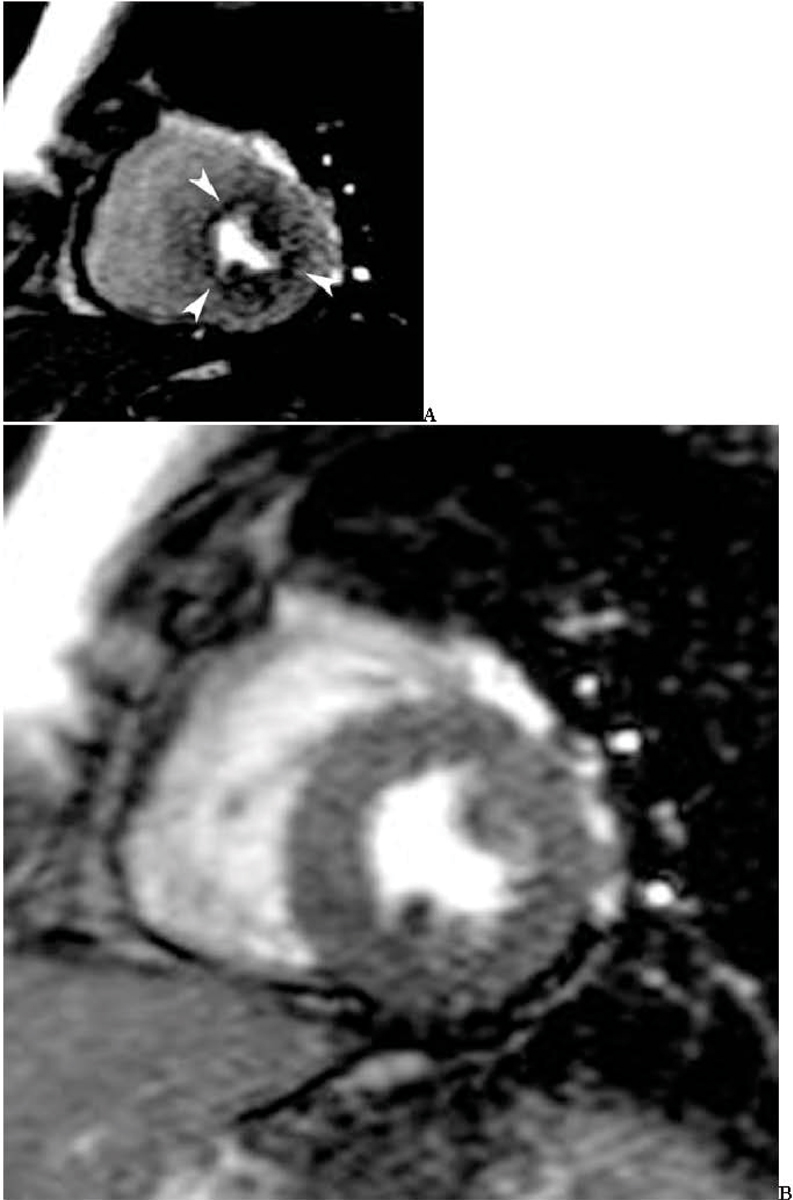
53 year old female patient with diabetes and hypertension, presenting to the emergency room with chest pain. (A) Diffuse subendocardial perfusion defect on adenosine stress MRI which was reversible at rest (B). The nuclear cardiac SPECT exam was normal, and there was no myocardial scar on delayed enhancement MRI.
